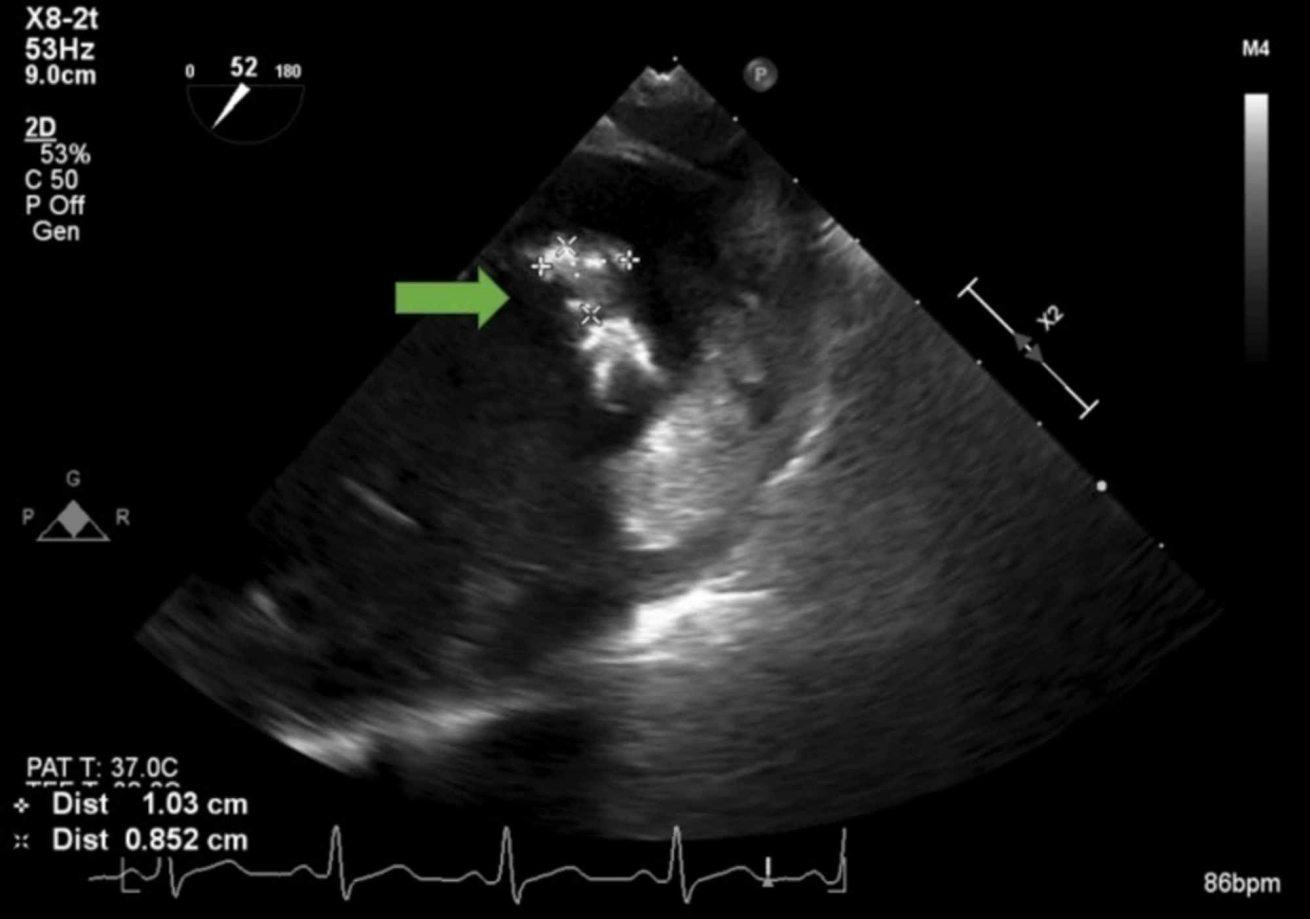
Transesophageal echocardiography showing mitral valve vegetation measuring 1 cm x 1 cm
